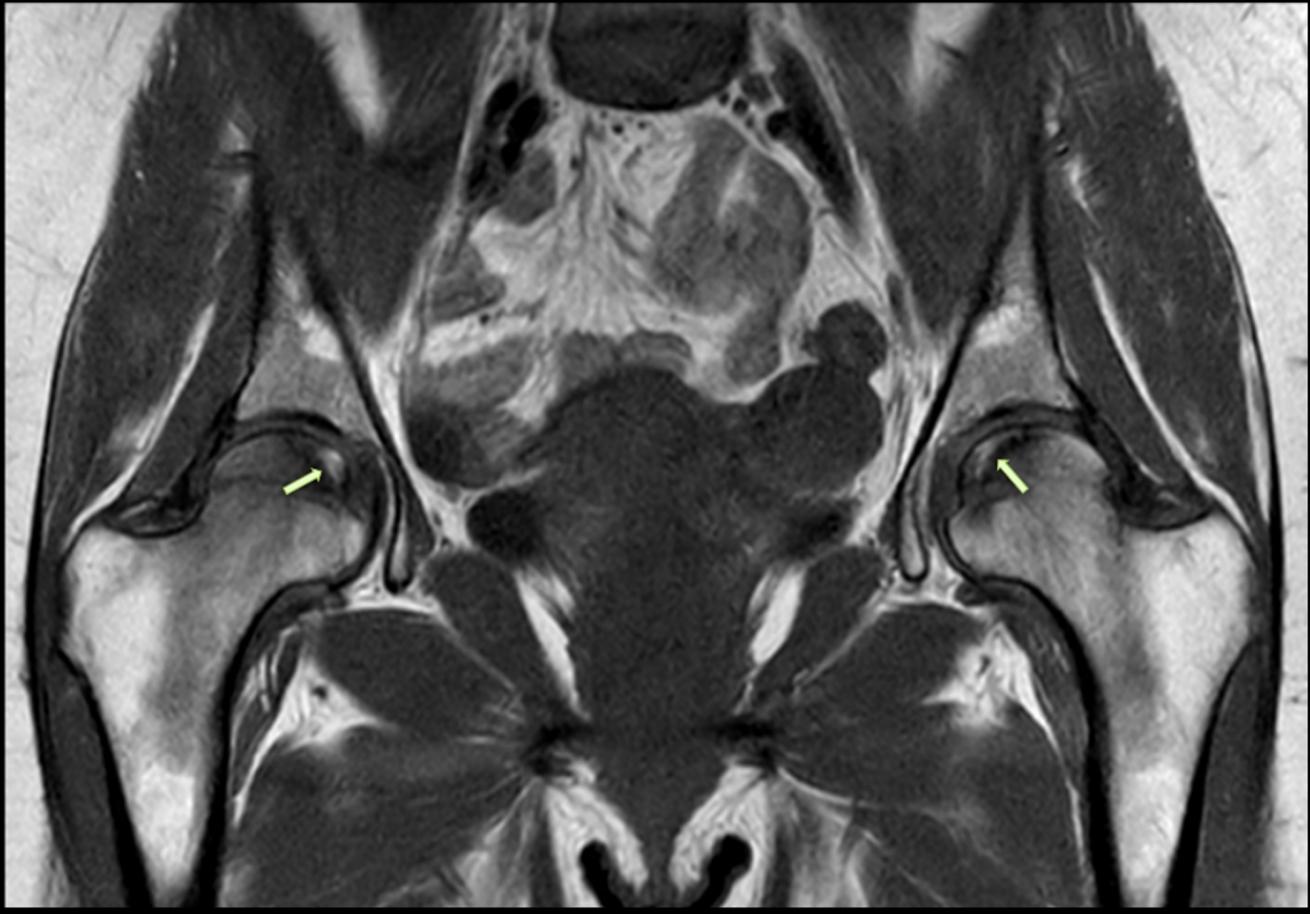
Coronal T2-weighted MRI of the pelvis showing bilateral AVNFH AVNFH: avascular necrosis of the femoral head; MRI: magnetic resonance imaging
